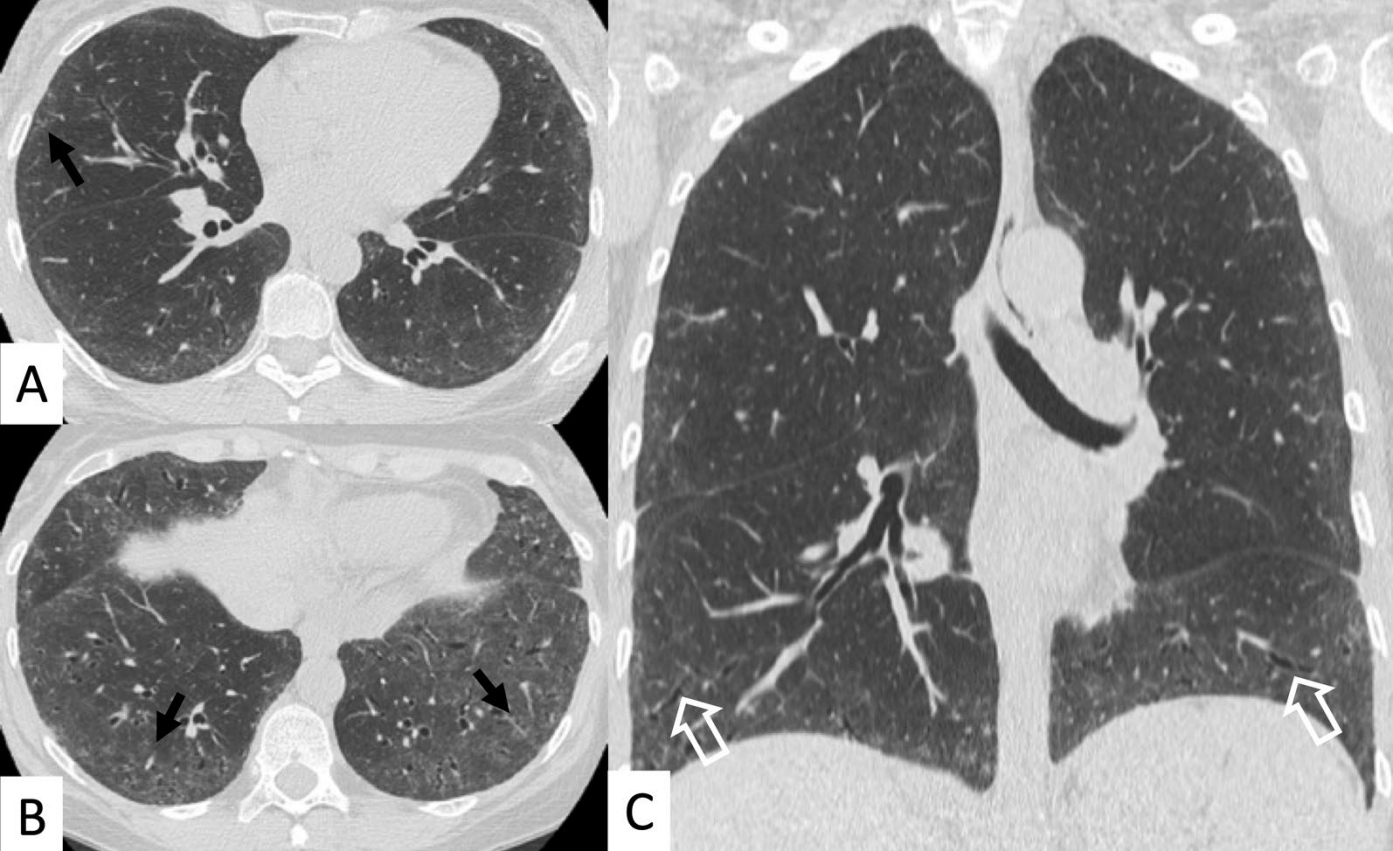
Axial (A, B) and coronal (C) HRCT images of an SSc patient demonstrate NSIP pattern with ground-glass opacities (black arrows), well depicted in the subpleural and basal regions of the lung; fine reticulations may be also observed. Coronal MPR image is useful to better delineate the presence of small bronchiectasis, which may be seen in the peripheral basal regions (white empty arrows)
